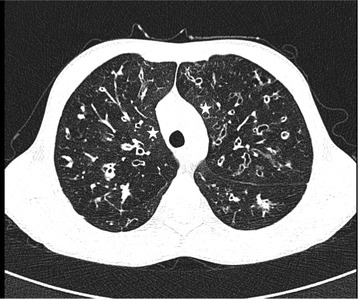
15 y/o male, CF-patient, FEV_1_ predicted 45%. Thin-section HR-reconstruction from MDCT (effective dose of 1.5 mSv). Severe bronchiectasis is visible. Note also dilated bronchi within the periphery of the lung. Air trapping is noted, only in central parenchyma, the CT attenuation appears normal (asterisks)
